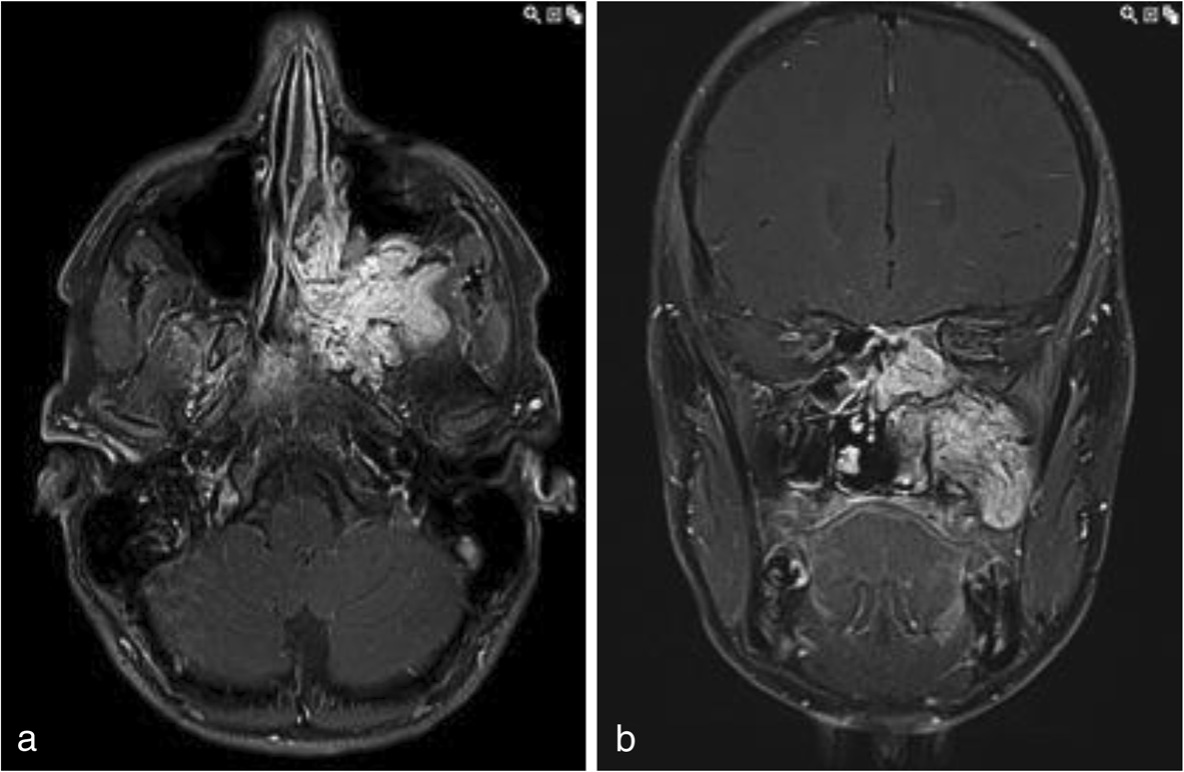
Preoperative MRI Scan of a 17-year-old boy with a juvenile nasopharyngeal angiofibroma: a shows the axial view and b shows the coronary scan
